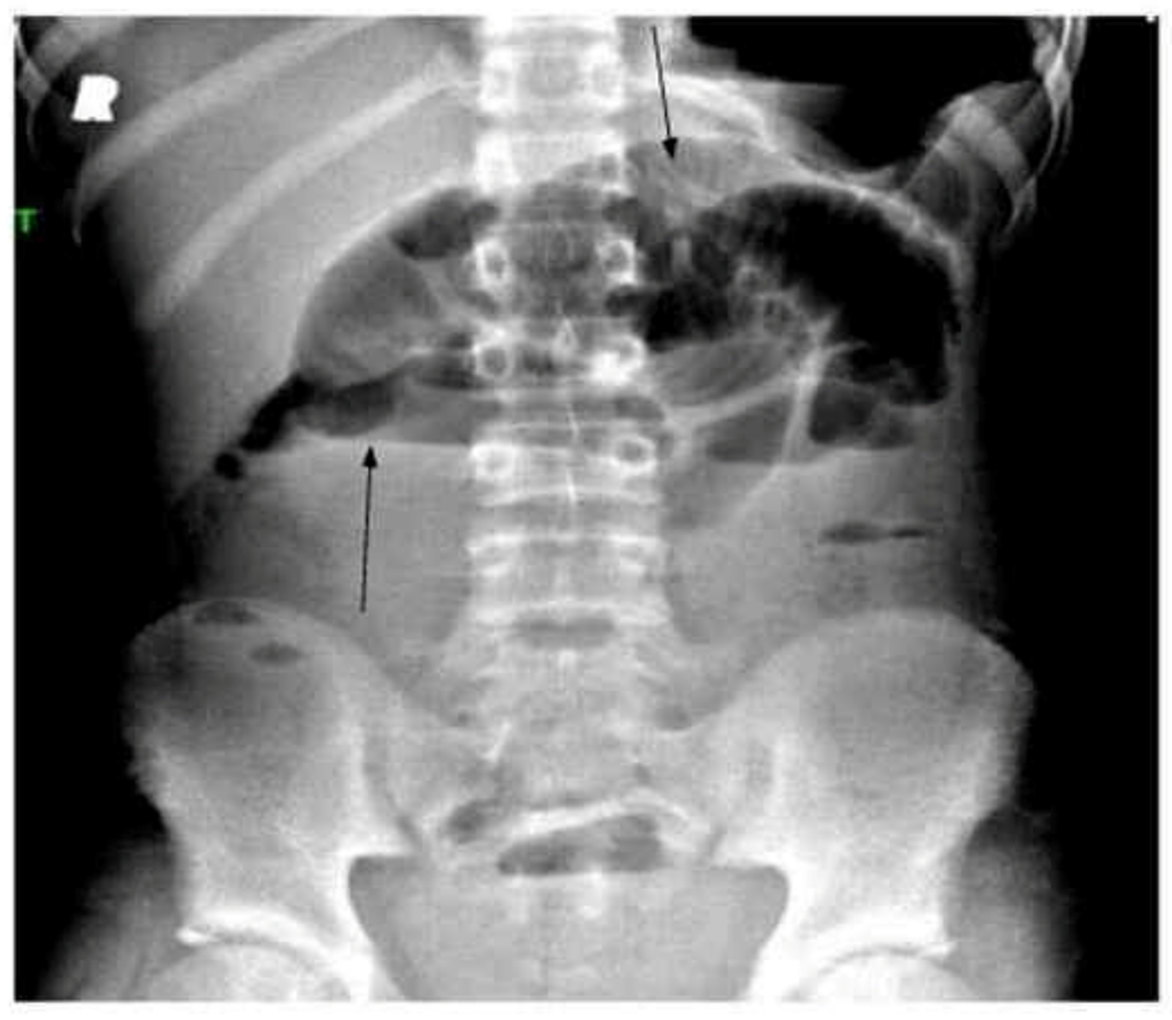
X-ray abdomen upright The image shows an abnormal bowel gas pattern with multiple abnormally dilated and air-filled loops of SB (black arrows). Partial distal SBO cannot be excluded although alternatively there may be an ileus SBO: small bowel obstruction
